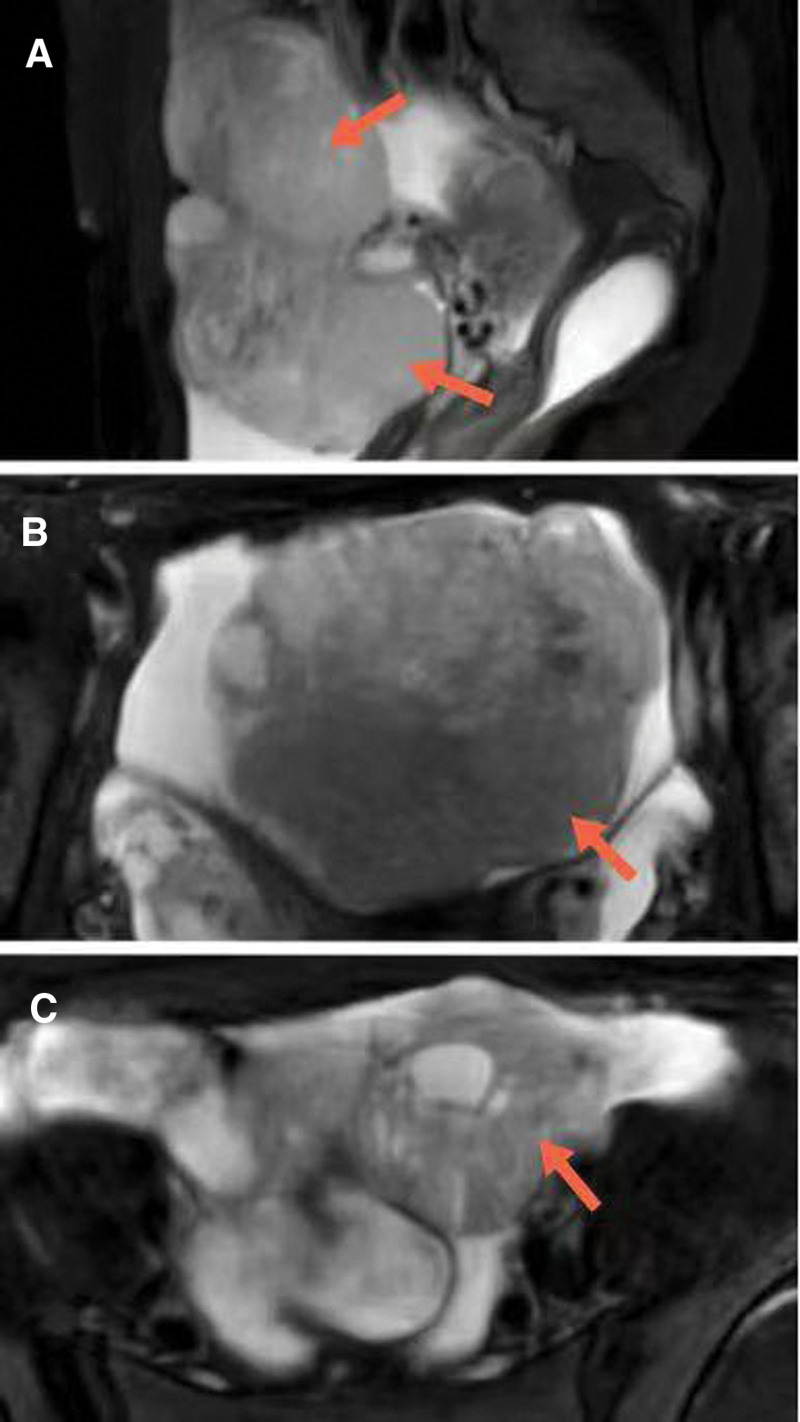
(A–C) A 36-year-old female patient with small cell carcinoma of the ovary, hypercalcemic type. Axial and sagittal T2-weighted MR images demonstrate a heterogeneous mass.
